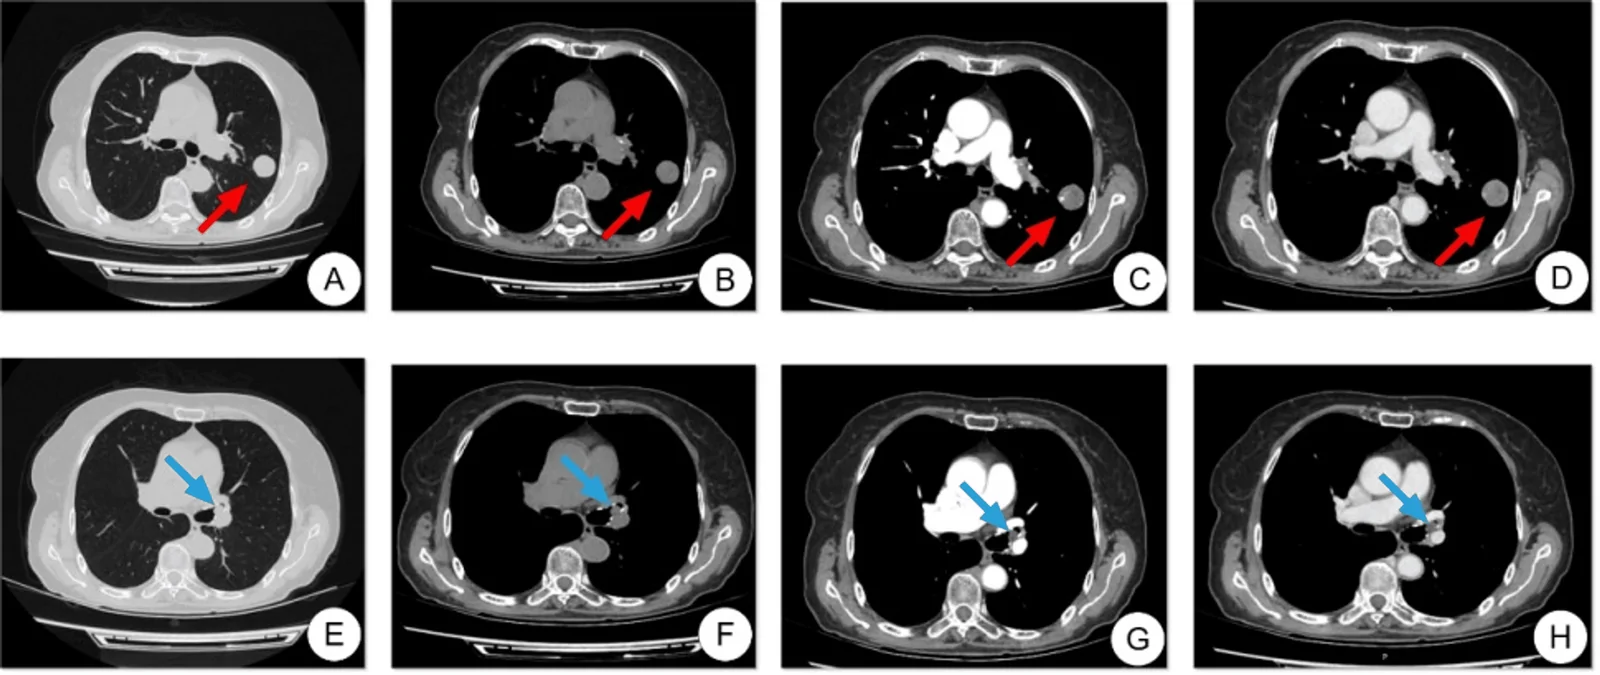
Contrast-Enhanced Chest Computed Tomography. (A-D) Lung-window, mediastinal-window, arterial-phase, and venous-phase images of the nodule in the apicoposterior segment of the left upper lobe. (E-H) Corresponding images of the endobronchial nodule in the left upper-lobe bronchus. The red arrow indicates an intrapulmonary lesion, and the blue arrow indicates an endobronchial lesion.
